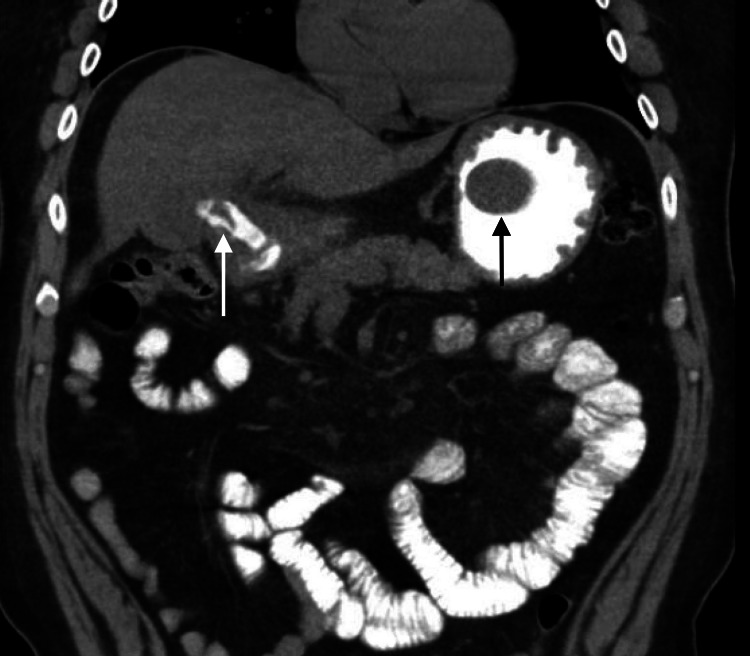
Coronal CT of the abdomen showing contrast crossing a cholecystoduodenal fistula (white arrow) and a 6 x 3-cm gallstone in the lumen of the stomach (black arrow)
